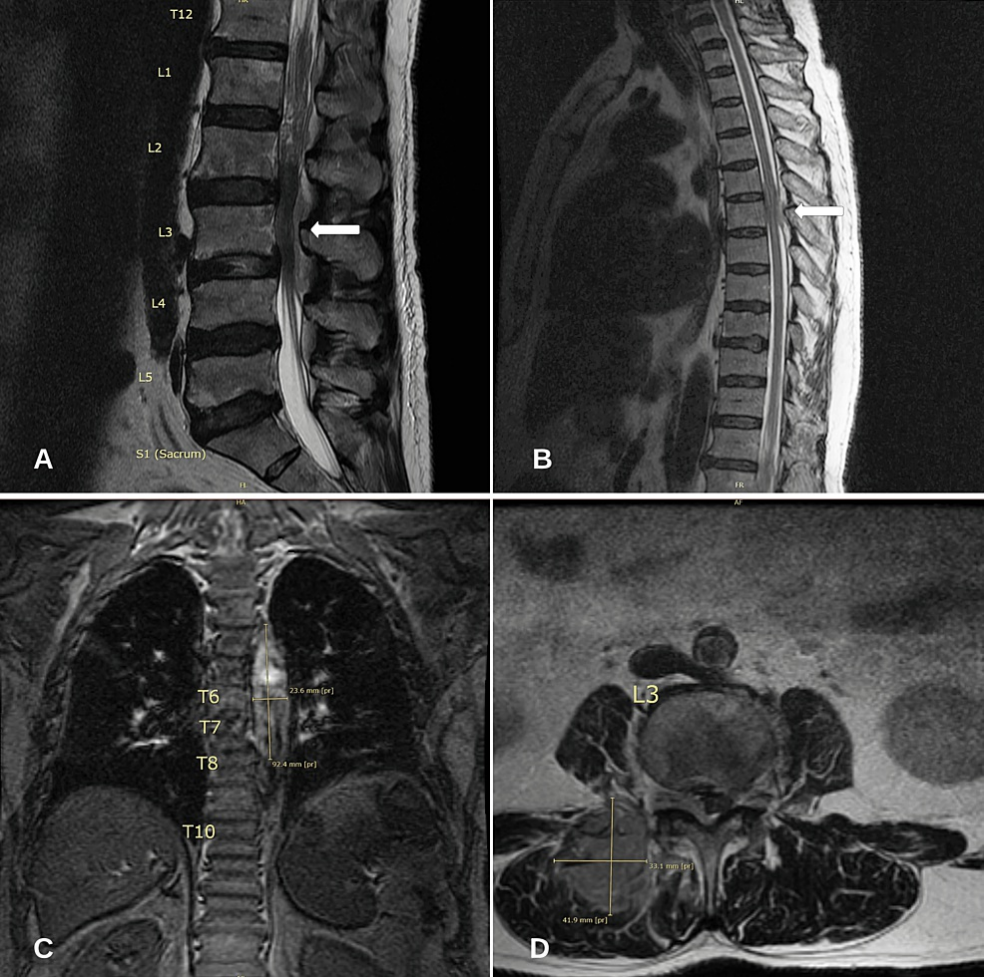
MRI of the thoracic and lumbar spine. (A) MRI T2 sagittal view of the lumbar spine revealing L2-L5 spinal canal stenosis. (B) MRI T2 sagittal view of the thoracic spine revealing significant cord compression at T5-T6 due to ventral and dorsal epidural masses. (C) MRI T2 coronal view of the thoracic spine revealing heterogeneous masses measuring 9.2 × 2.3 cm likely representing metastatic disease. (D) MRI T2 axial view of the lumbar spine revealing a mass measuring approximately 3.1 × 4.1 cm in the right paraspinal musculature with direct epidural extension and neural foraminal narrowing. MRI: magnetic resonance imaging
